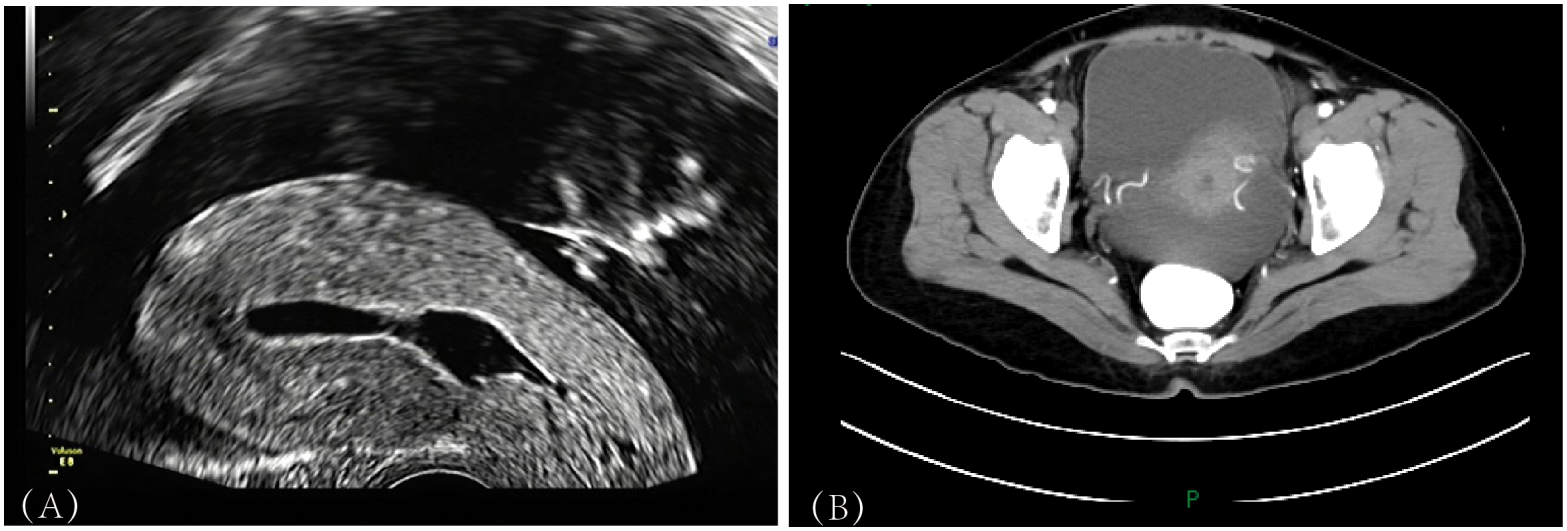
(A) Transvaginal ultrasound image showing a hyperechoic lesion within the endocervical canal. (B) Abdominal contrast-enhanced CT scan clearly demonstrating a large cystic-solid mass encasing both adnexa, accompanied by ascites.
